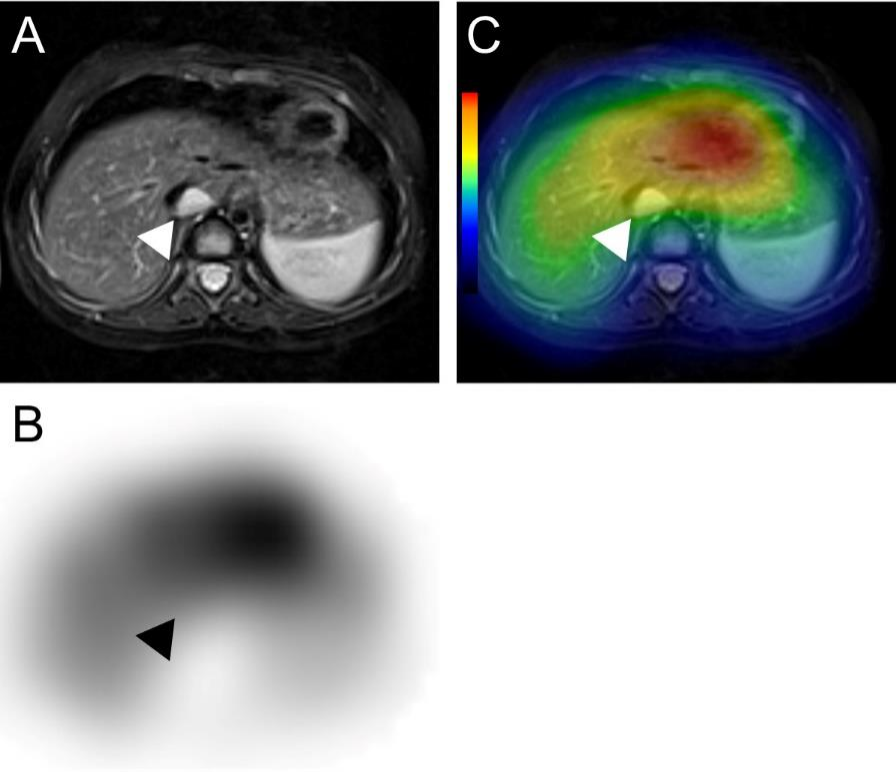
Fat suppressed T2-weighted MRI visualized a small mass behind the inferior vena cava (A). Its uptake was equal to the liver on I-123 MIBG scintigraphy (B-C)
